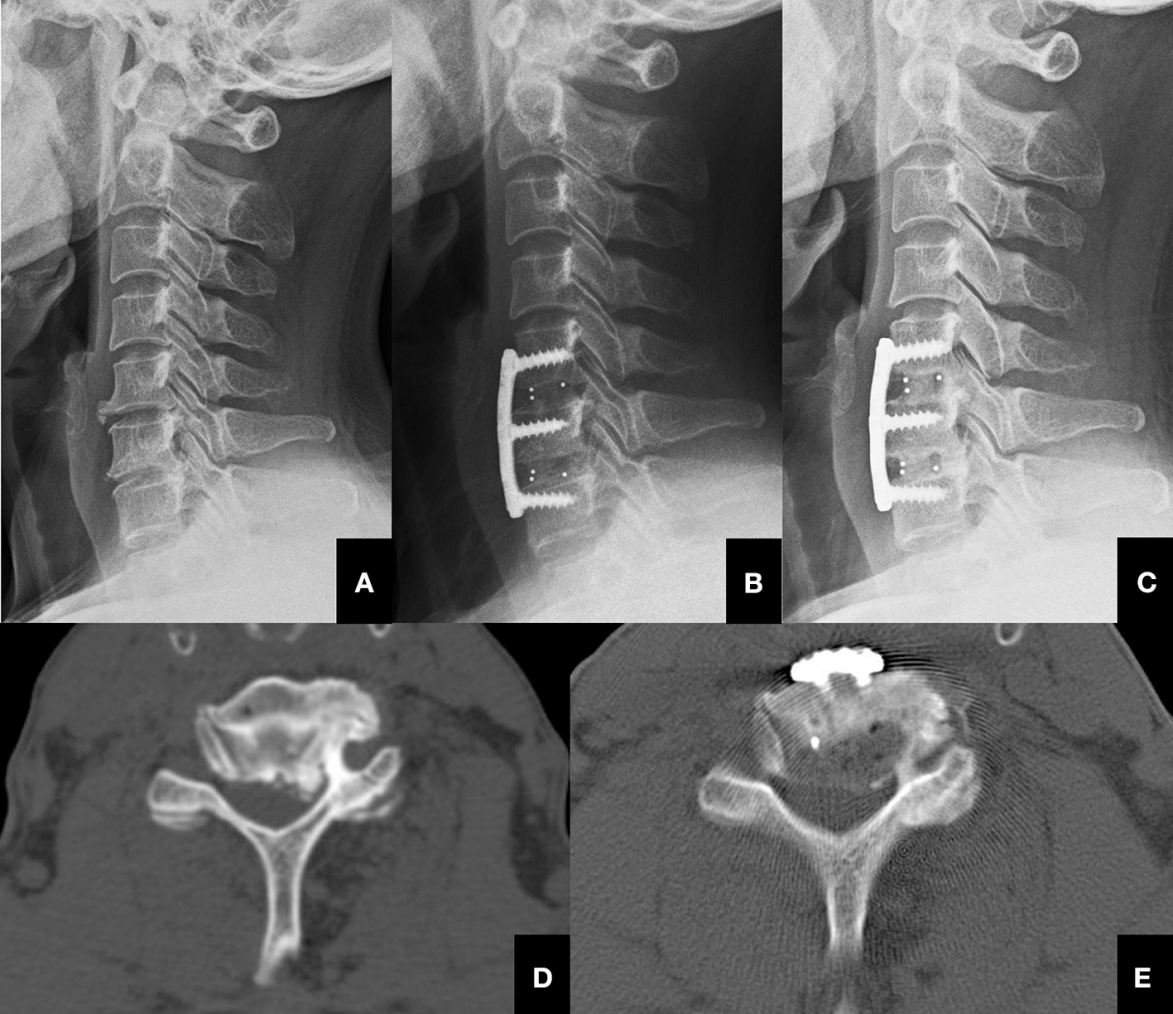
The process of performing UPR. (A) Typical lateral pre-operative X-ray image of a 56-year-old female patient with cervical radiculopathy ready to receive ACDF + UPR treatment. (B) Post-operative lateral X-ray image of the patient that received ACDF + UPR treatment. (C) One-year post-operative lateral X-ray image of the patient that received ACDF + UPR treatment, and showed spinal fusion was achieved and cervical alignment was sustained. (D) Pre-operative CT image showing severe hypertrophied left uncinate process of C5-C6 level. (E) Post-operative CT image showing the resected uncinate process of C5-C6 level.
